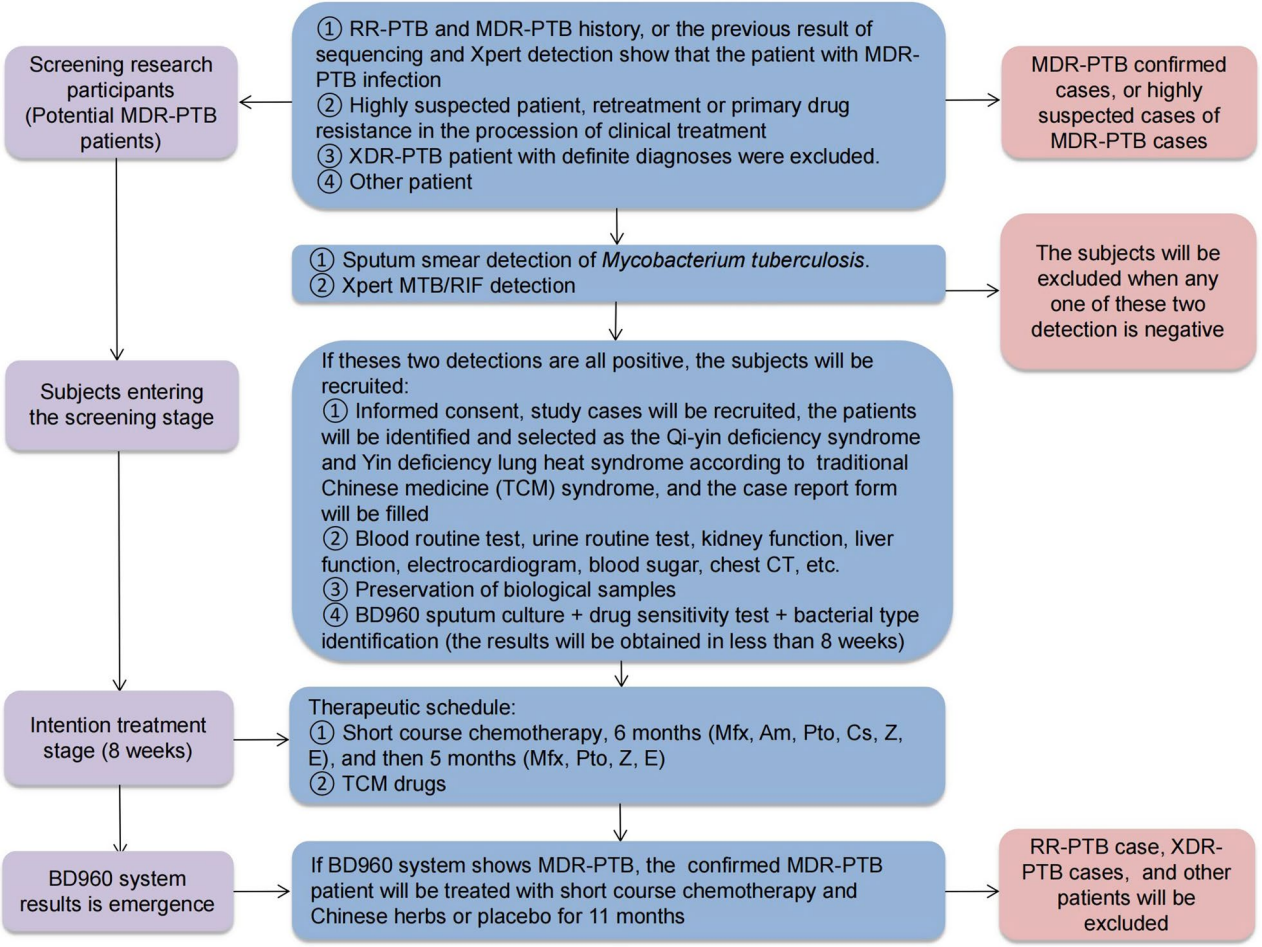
Initial screening process for patients in present study. *Am* Amikacin; *Cs* Cycloserine; *CT* Computed tomography; *E* Ethambutol (or EMB); *MDR-PTB* Multi-drug resistant pulmonary tuberculosis; *Mfx* Moxifloxacin; *Pto* Prothionamide; *RR-PTB* Rifampicin resistant pulmonary tuberculosis; *TCM* Traditional Chinese medicine; *XDR-PTB* Extensively drug-resistance pulmonary tuberculosi; *Z* Pyrazinamide (or PZA)
